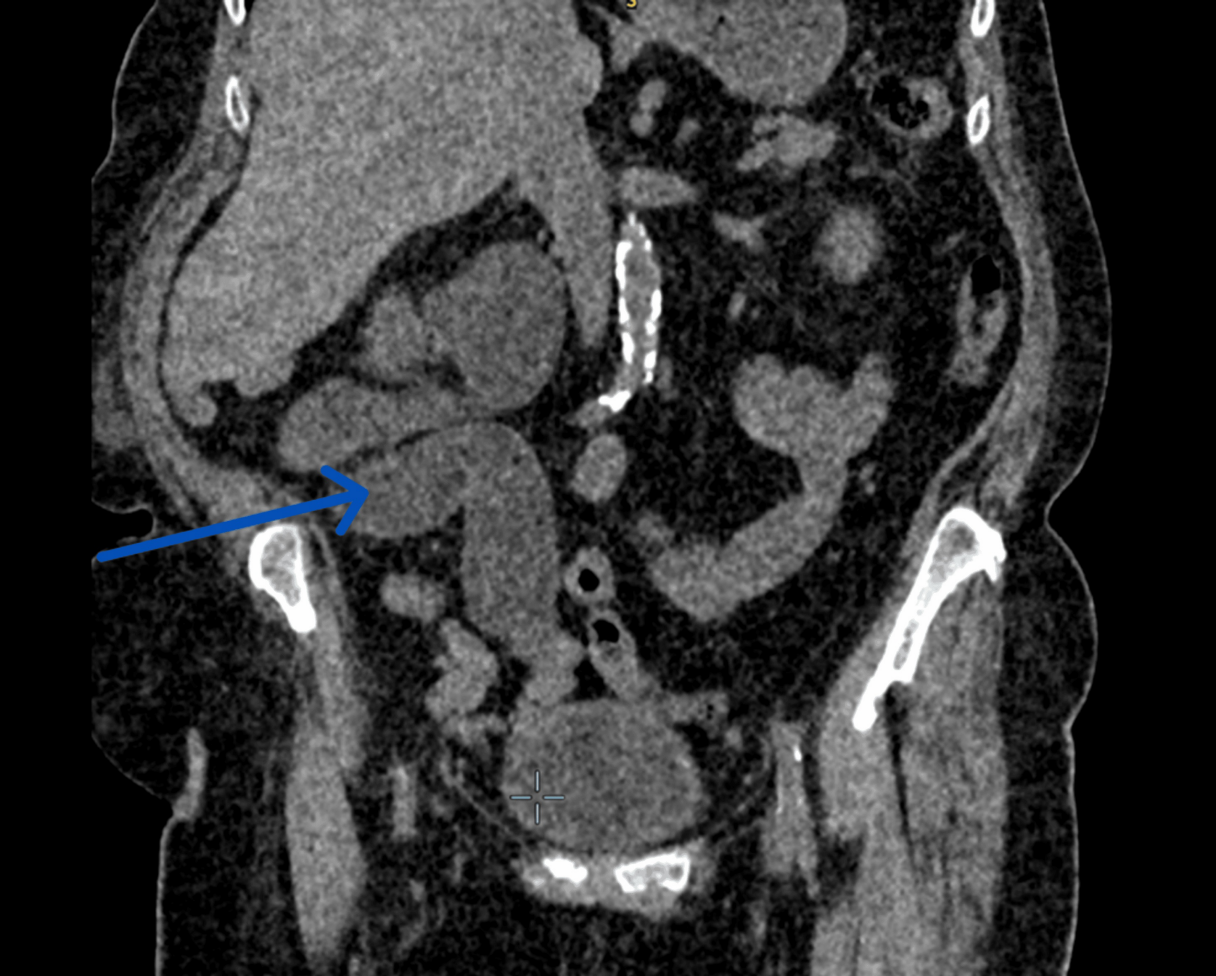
New CT coronal section The blue arrow shows a worsening, tortuous, dilated right ureter.
